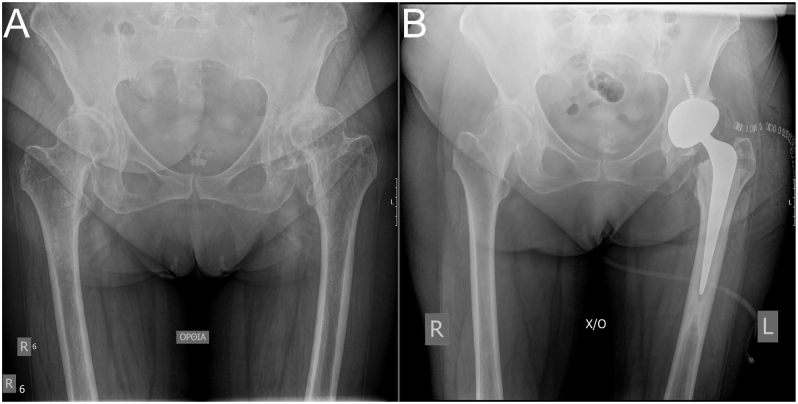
Preoperative (A) and immediate postoperative (B) radiographs of a Hartofilakidis B1 developmental dysplasia of the left hip treated with hybrid total hip arthroplasty using a cemented Exeter stem.
